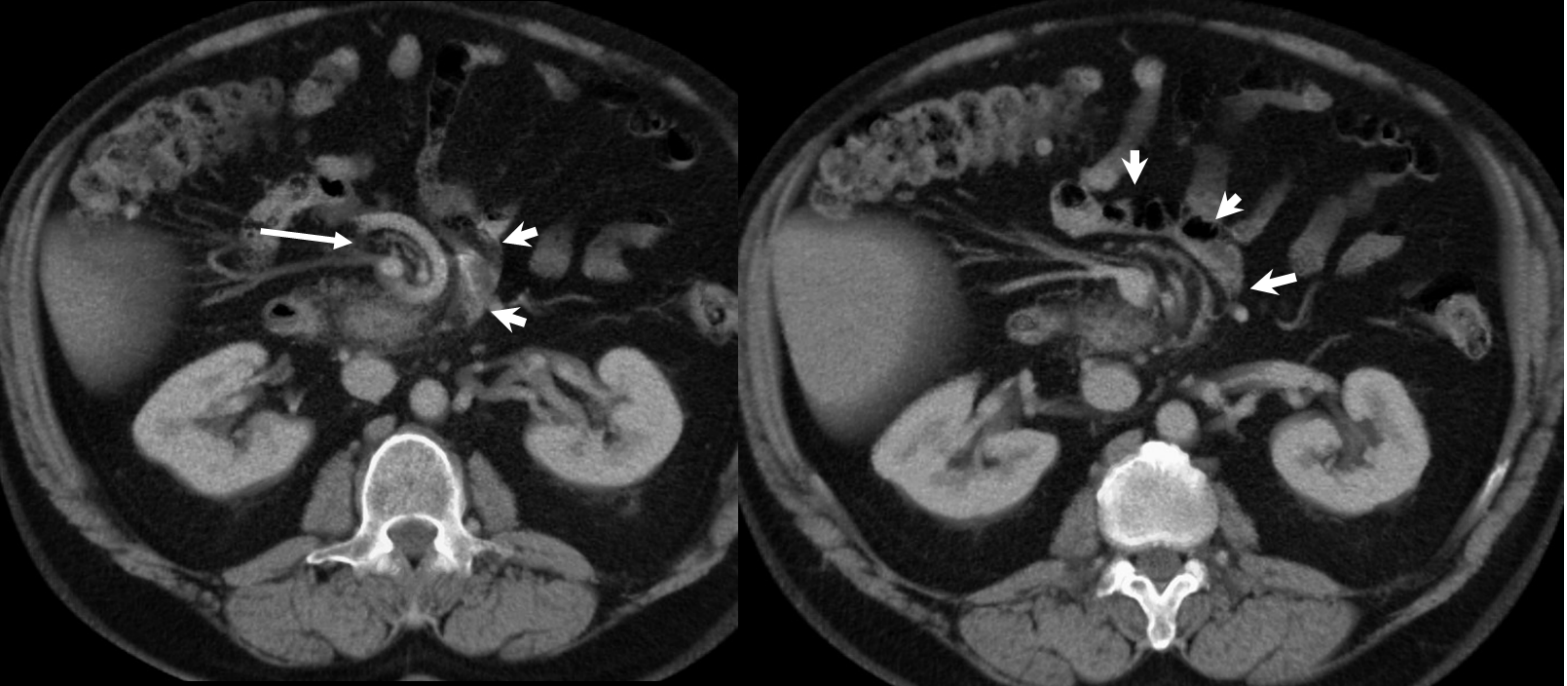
Contrast enhanced CT axial images at the level of L2 demonstrating abnormal rotation of the proximal jejunum (short arrows). Note the swirling of the superior mesenteric vein (long arrow).
